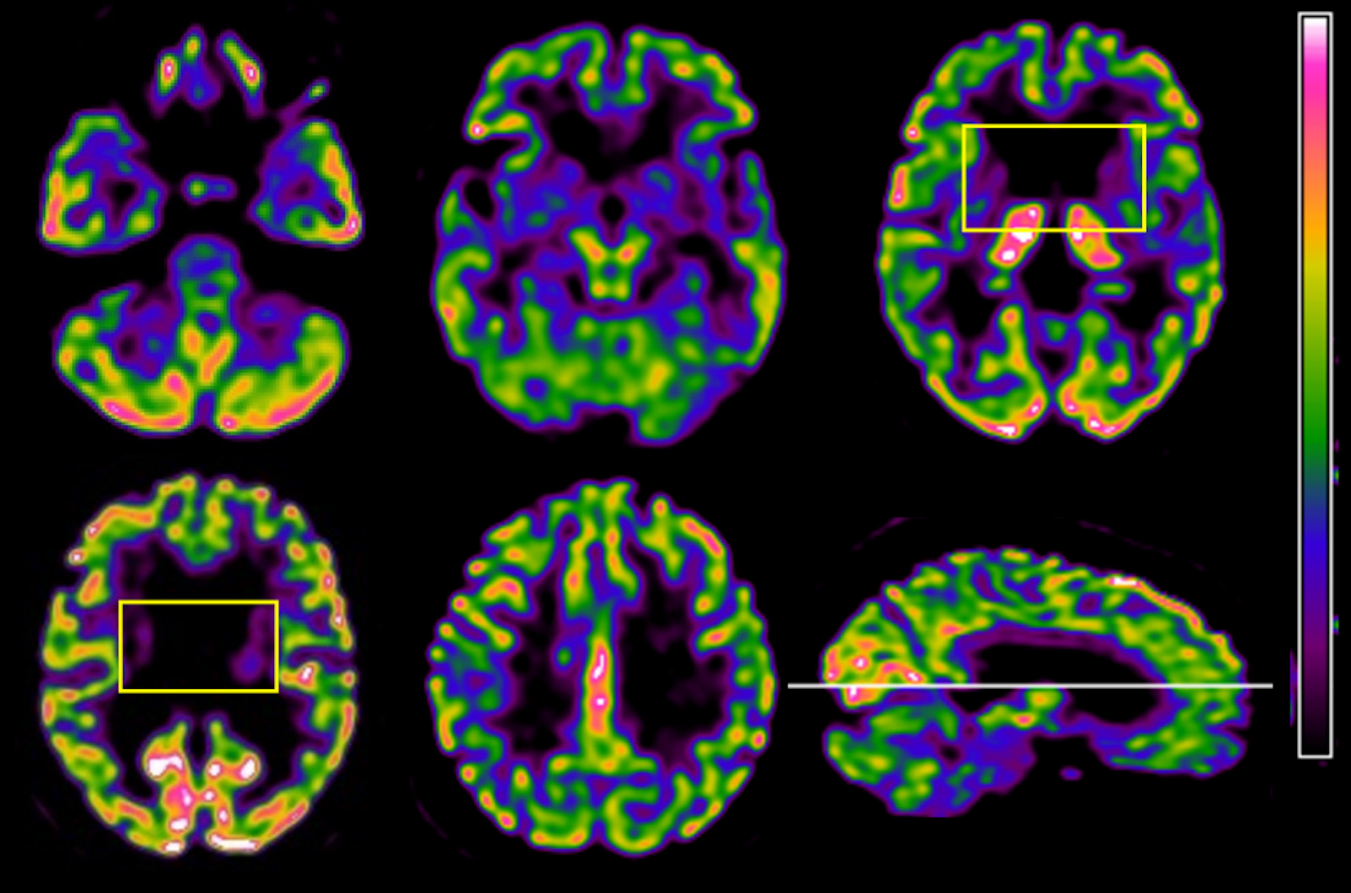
18F‐Fluorodeoxyglucose (FDG) PET images showing severe hypometabolism in bilateral basal ganglia (outlined boxes), but symmetrically preserved metabolism in midbrain, cerebellum, bilateral thalamic nuclei, and cortical areas
